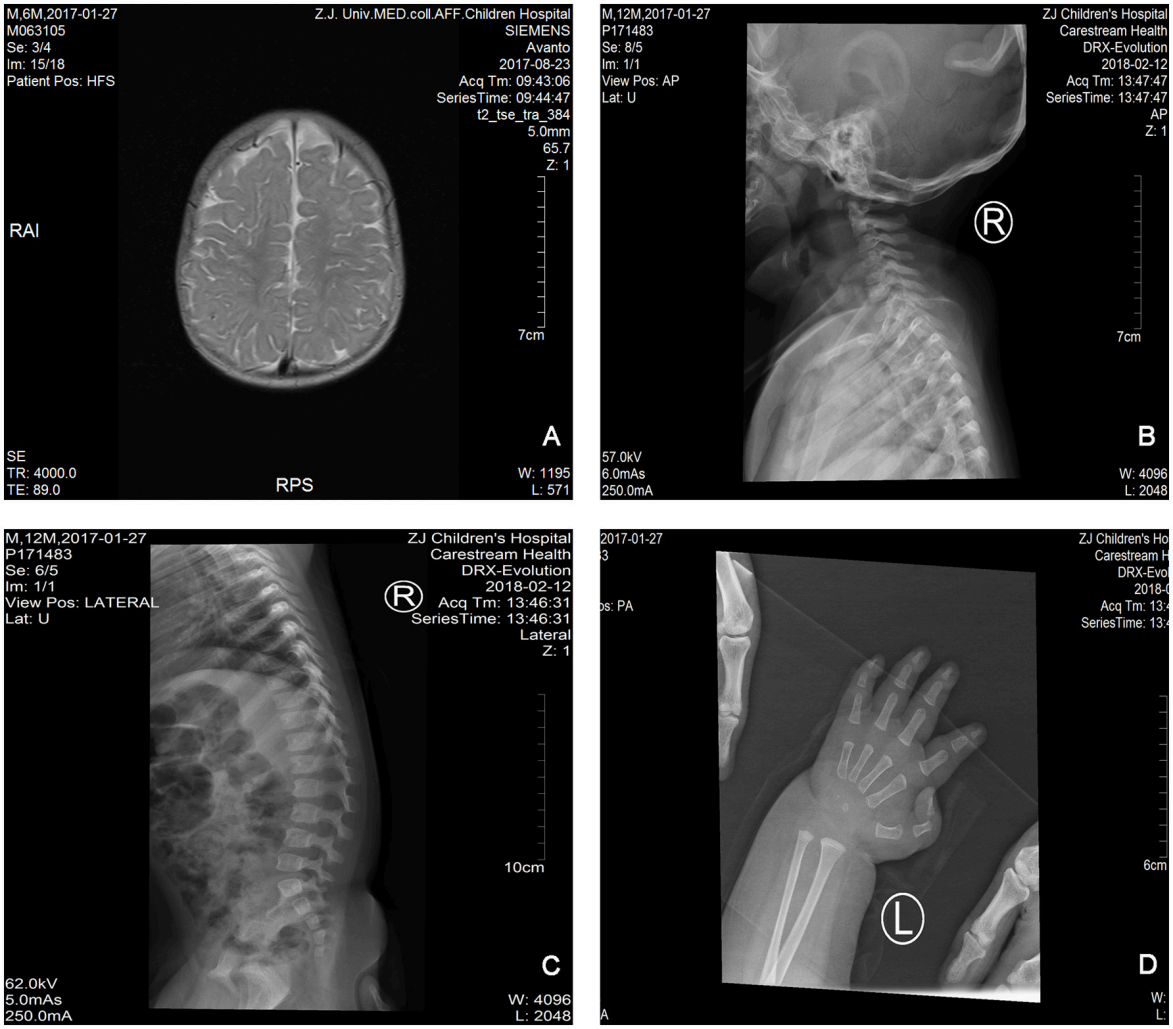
The magnetic resonance imaging (MRI) and X-ray images of the Coffin–Lowry syndrome case. (A) The MRI of cerebral development; (B,C) Cervical and spinal canal X-ray photographs. (D) Nodular hyperplasia of finger tail end.
